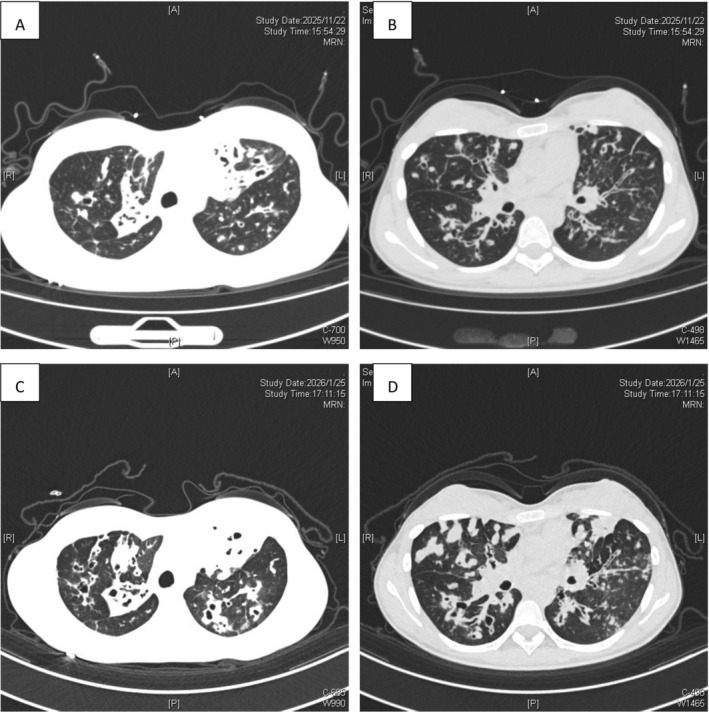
HRCT comparison between the previous (A, B) and current (C, D) hospitalizations. Panels (A, C) and (B, D) represent matched anatomical levels, respectively. The current images show marked progression of bilateral bronchiectasis, mucus plugging, and pulmonary consolidation, consistent with aggravated pulmonary infection.
